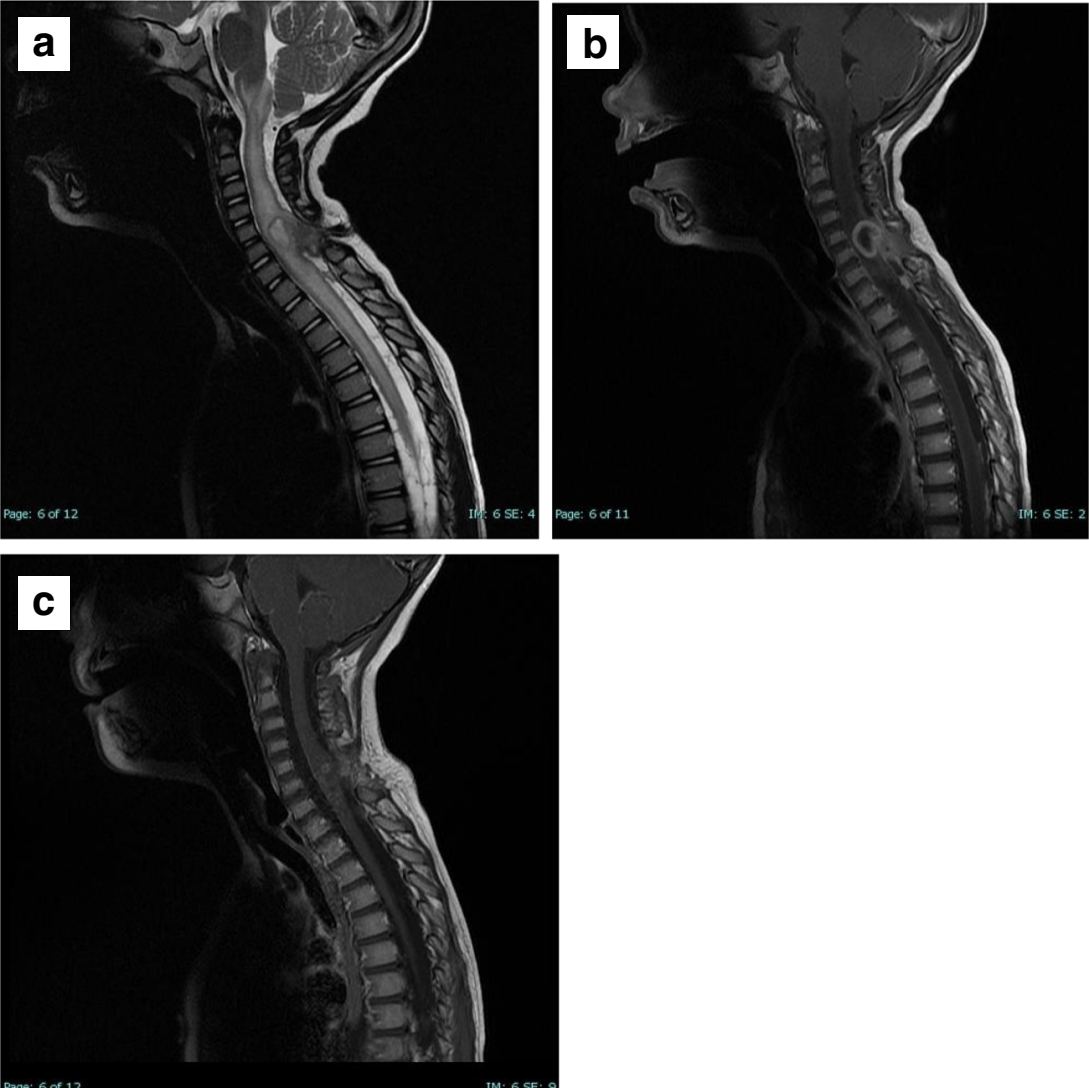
Preoperative sagittal MRI of the spine. a T2-weighted image demonstrating a dermal sinus tract at C6, connecting the skin to spinal cord and associated oedema extending from the medulla to T9. b Preoperative T1-weighted MRI with contrast showing an abscess at C5-C6. c Post-operative T1-weighted MRI with contrast at 6 months showing complete removal of dermal sinus tract and resolution of the abscess.
